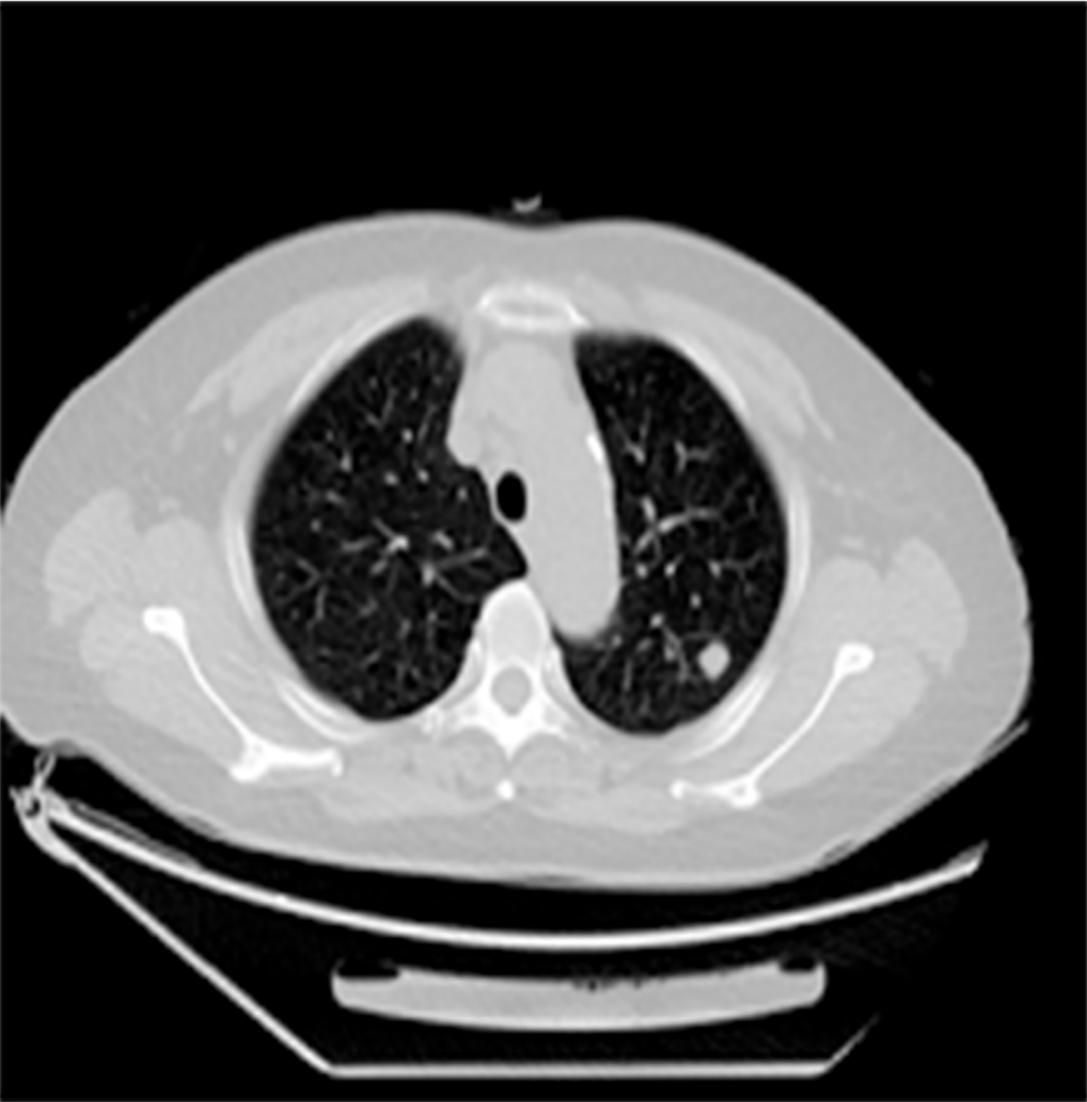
Chest CT showed that a soft tissue nodule in the left upper lobe with lobulated contours, inhomogeneous density, and calcifications is visible inside, and the largest cross-sectional dimension of the mass was 1.0 cm × 1.1 cm.
